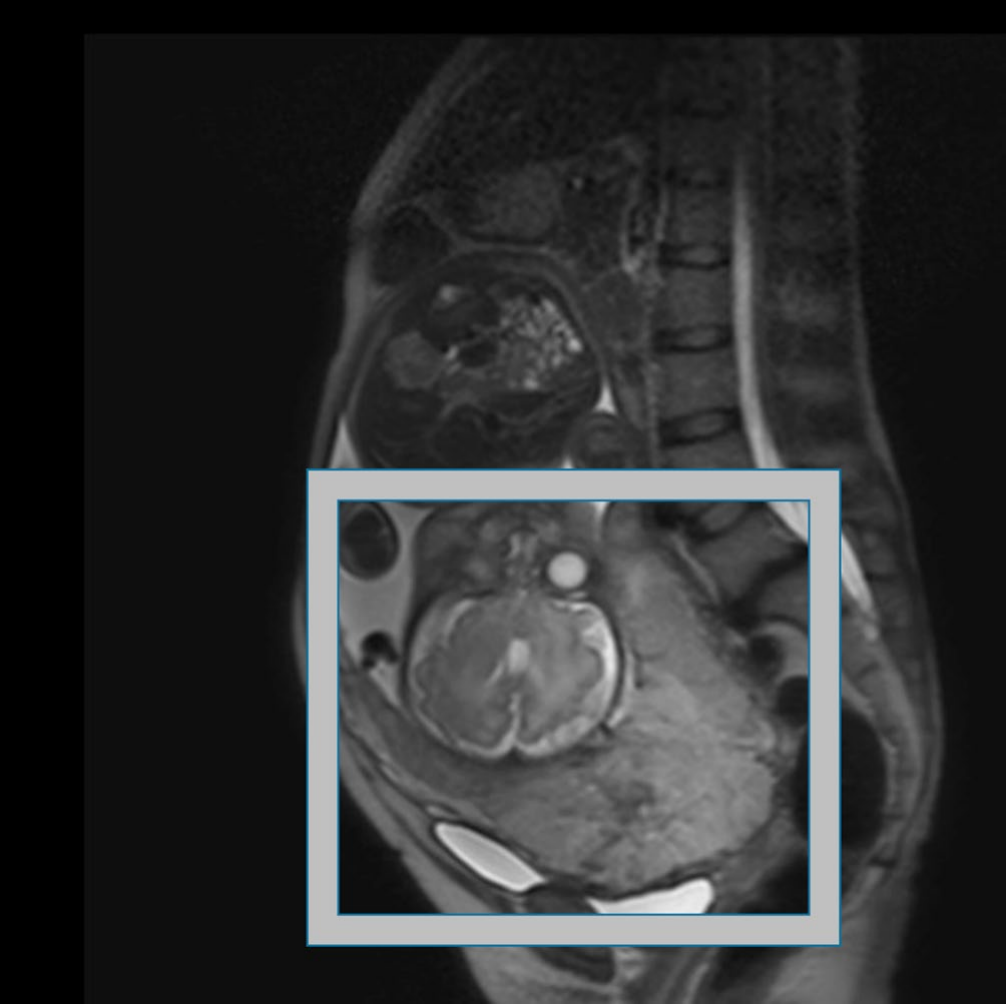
An example of MRI image of placenta previa. In this case, the placenta covered the anterior wall and os of the uterus, which was considered to cause massive bleeding during cesarean section. However, in this case, the blood loss was under 2000 ml. In the model construction, the field shown by the white bounding box was used for the learning.
